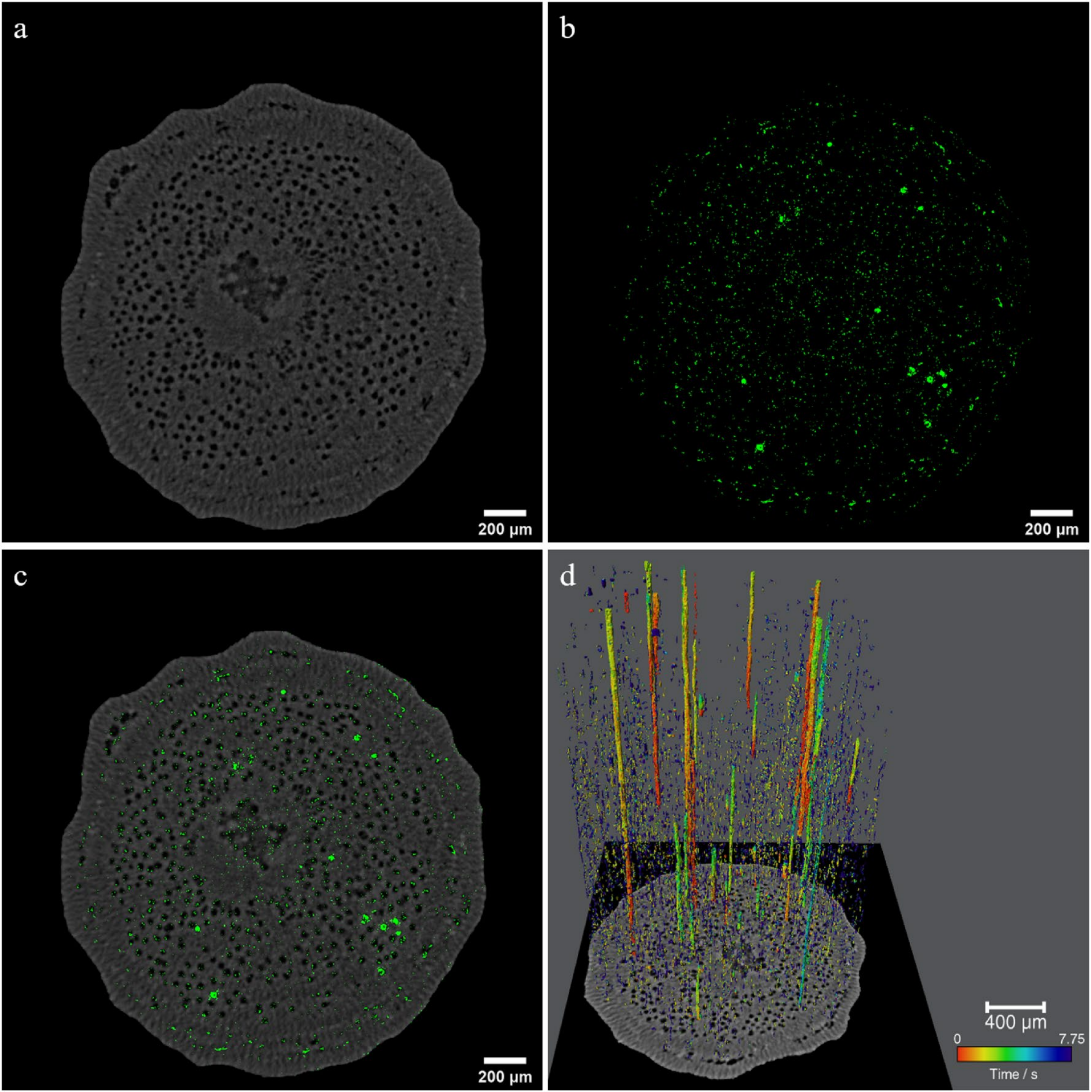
MCXCT results for willow branch water refilling. (a) CT slice No. 500 of the willow branch. (b) MCXCT slice No. 500 at 7.75 s. (c) Fusion of panels (a) and (b). (d) 3D dynamic spatiotemporal distribution of willow branch water refilling obtained from MCXCT.
